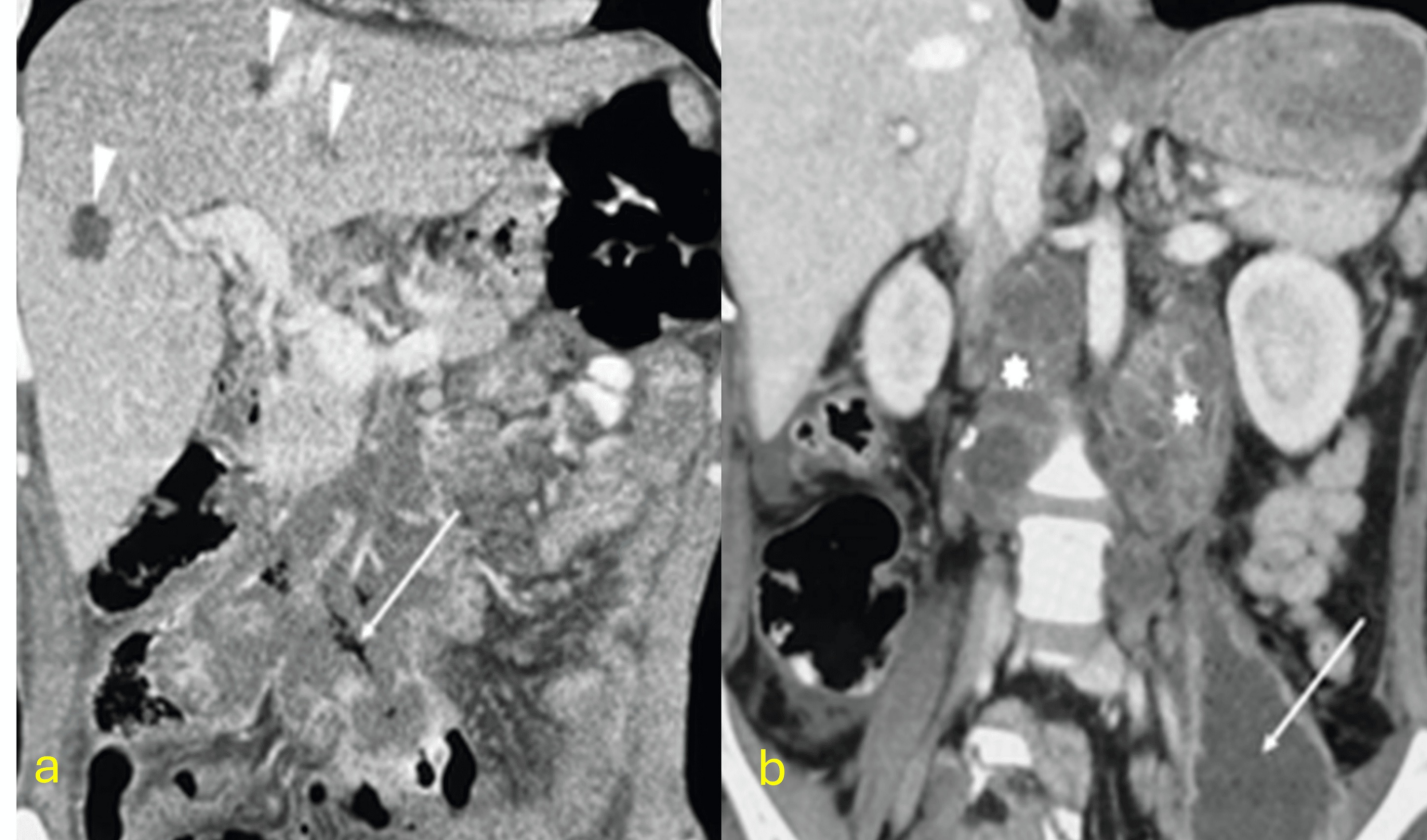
Abscessed lymph node tuberculosis CT: computed tomography (a) Coronal contrast-enhanced CT reconstructions showing mesenteric adenopathy conglomerates with hypodense areas and rim enhancement. Air bubbles indicate a fistula to the colon (arrow). Hepatic abscesses are also noted (arrowhead). (b) Retroperitoneal adenopathy (asterisks) compromising the left psoas muscle with abscess formation. Diagnosis confirmed by CT-guided biopsy of mesenteric lymph nodes showing granulomatous inflammation with caseation and positive GeneXpert MTB/RIF
